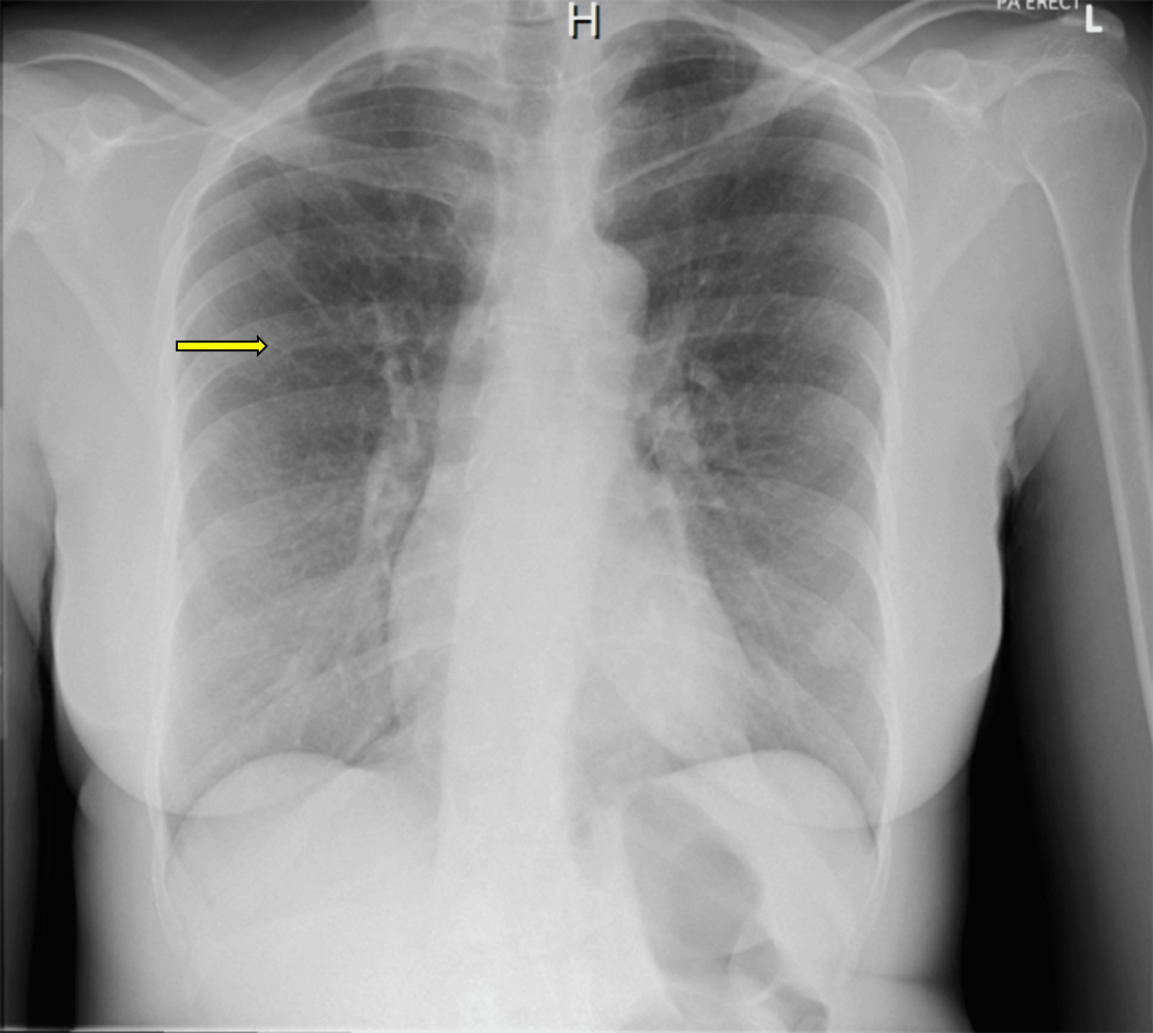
Chest X-ray showing miliary nodules and a tree-in-bud appearance (arrow).
